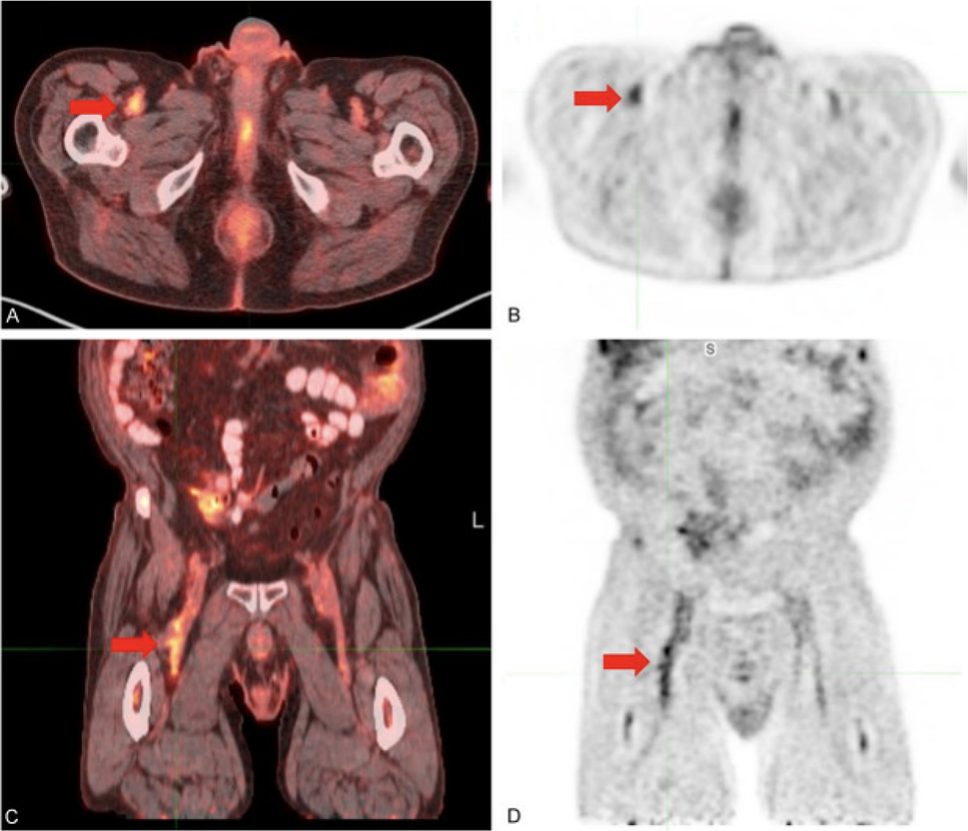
Venous thrombosis in the right common femoral vein, diagnosed prior to the ultrasound. A Axial FDG-PET/CT, B axial FDG-PET, C coronal FDG-PET/CT, and D coronal FDG-PET demonstrated high metabolic activity in the lumen of the right common femoral vein, consistent with venous thrombosis. Ultrasound performed two weeks later confirmed non-occlusive thrombus in the right common femoral vein (Reproduced from Kaghazchi et al. [69] under open copyright policy of the journal)
